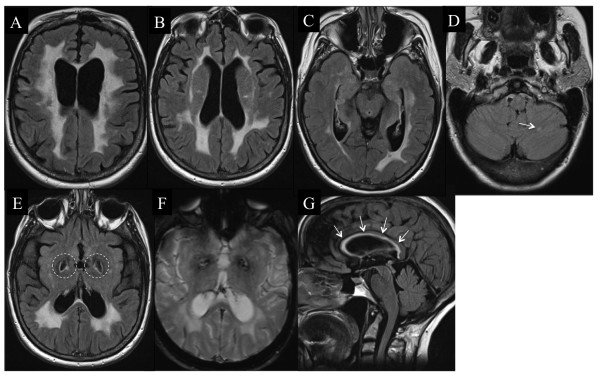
Brain and spinal cord MRI of patient 2. Flair sequence showing brain abnormalities typical of CADASIL (A) including the external capsule (B) but with numerous atypical findings for CADASIL: absence of temporal lobe involvement (C), cerebellar lesion in the left hemisphere (D, arrow), large bilateral cavities in the pallidum (E, dotted circles) without any microbleeds on T2*-weighted sequences (F), and a lesion in the corpus callosum, which has been rarely described in CADASIL (G, arrows).
